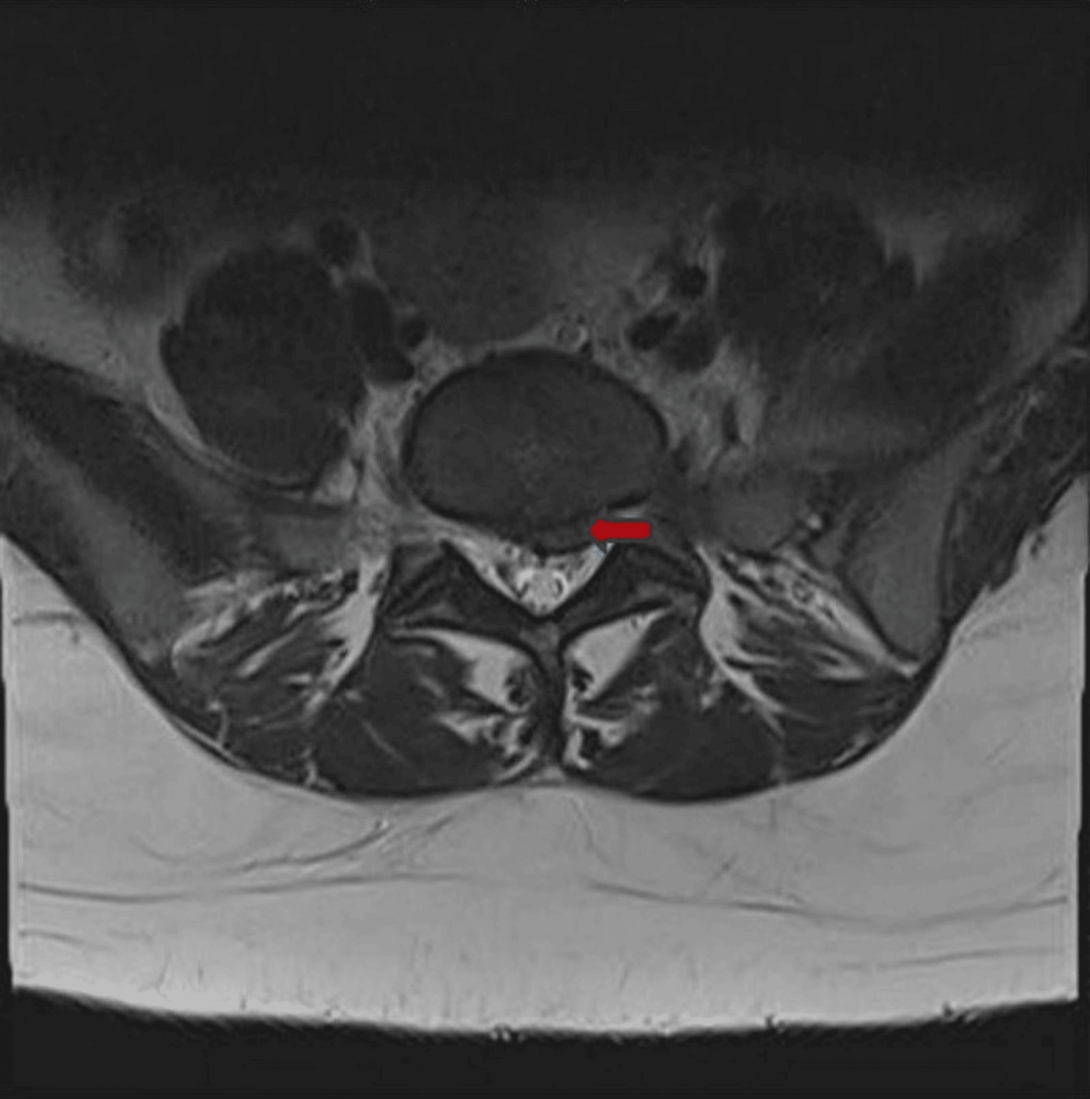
MRI of lumbar spine on admission showing left paracentral disc herniation.
